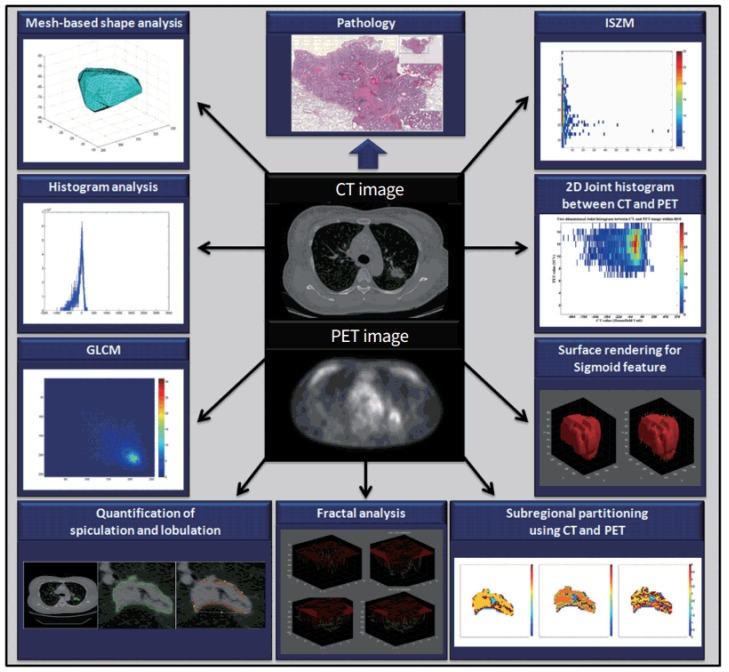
Examples of various radiomics features that can be extracted from CT and positron emission tomography (PET) images. Gray-level co-occurrence matrix (GLCM), histogram analysis, mesh-based shape, intensity size zone matrix (ISZM), two-dimensional joint histogram, surface rendering for sigmoid features, and sub-regional portioning are various features than can be extracted from CT and PET images. These features can then be correlated with clinicopathologic data to gain prognostic and predictive information. Reproduced under a CC-BY-NC 4.0 license from [19].
